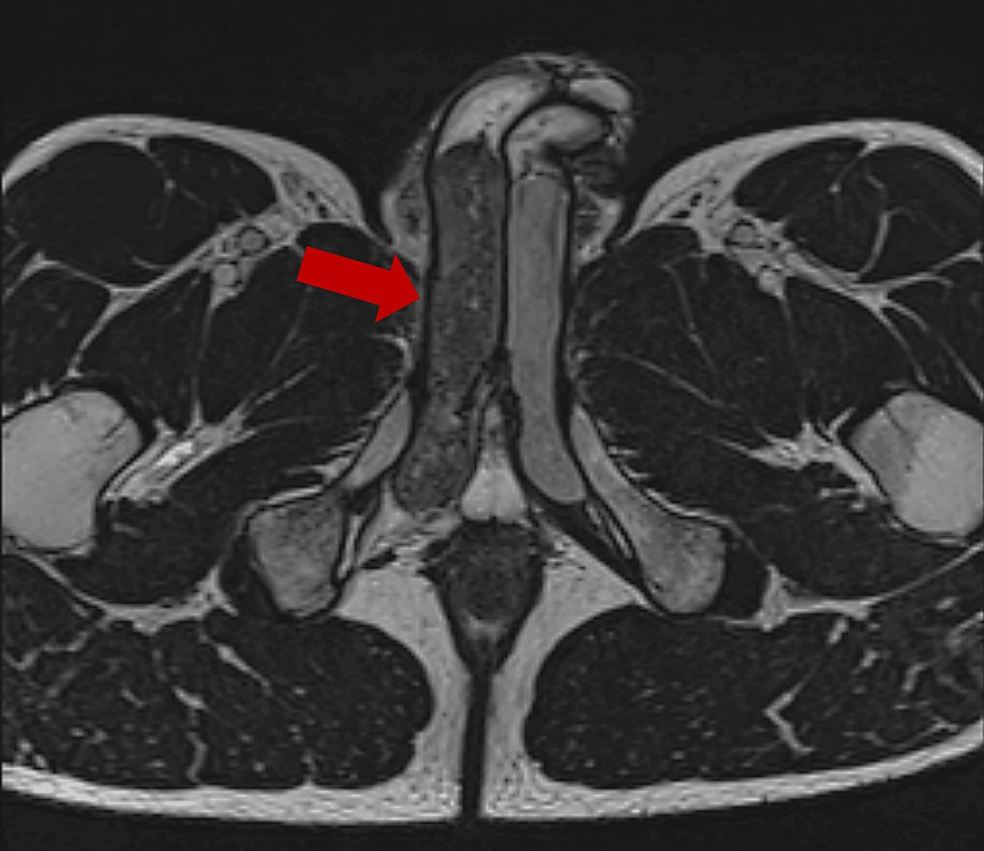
Transverse T2-weighted MRI. Enlarged appearance of the proximal portion of the right corpus cavernosum compared to the left with hypointense T2 signal intensity. A red arrow point at the right corpus cavernosum.
